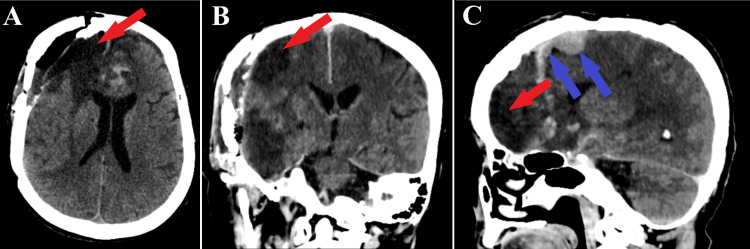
Postoperative CT scan. A: Axial section of the tissular CT window shows the complete tumor resection (red arrow).
